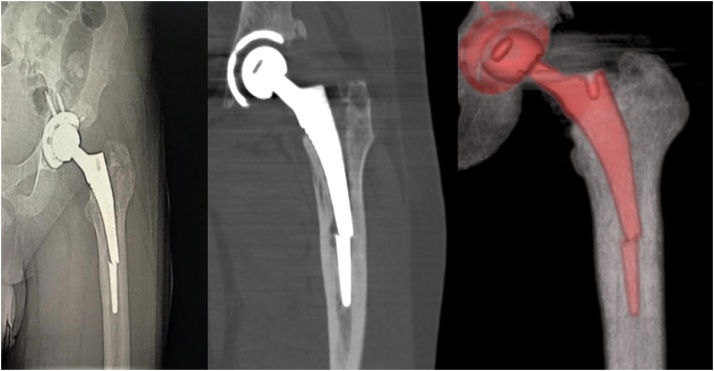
X-rays and CT scans of the hip 18 months after primary total hip arthroplasty.
